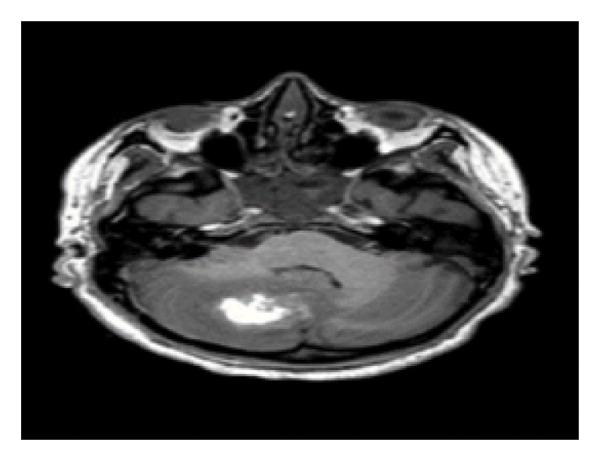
Axial MRI brain (T2‐weighted/FLAIR) showing a cerebellar hematoma.
